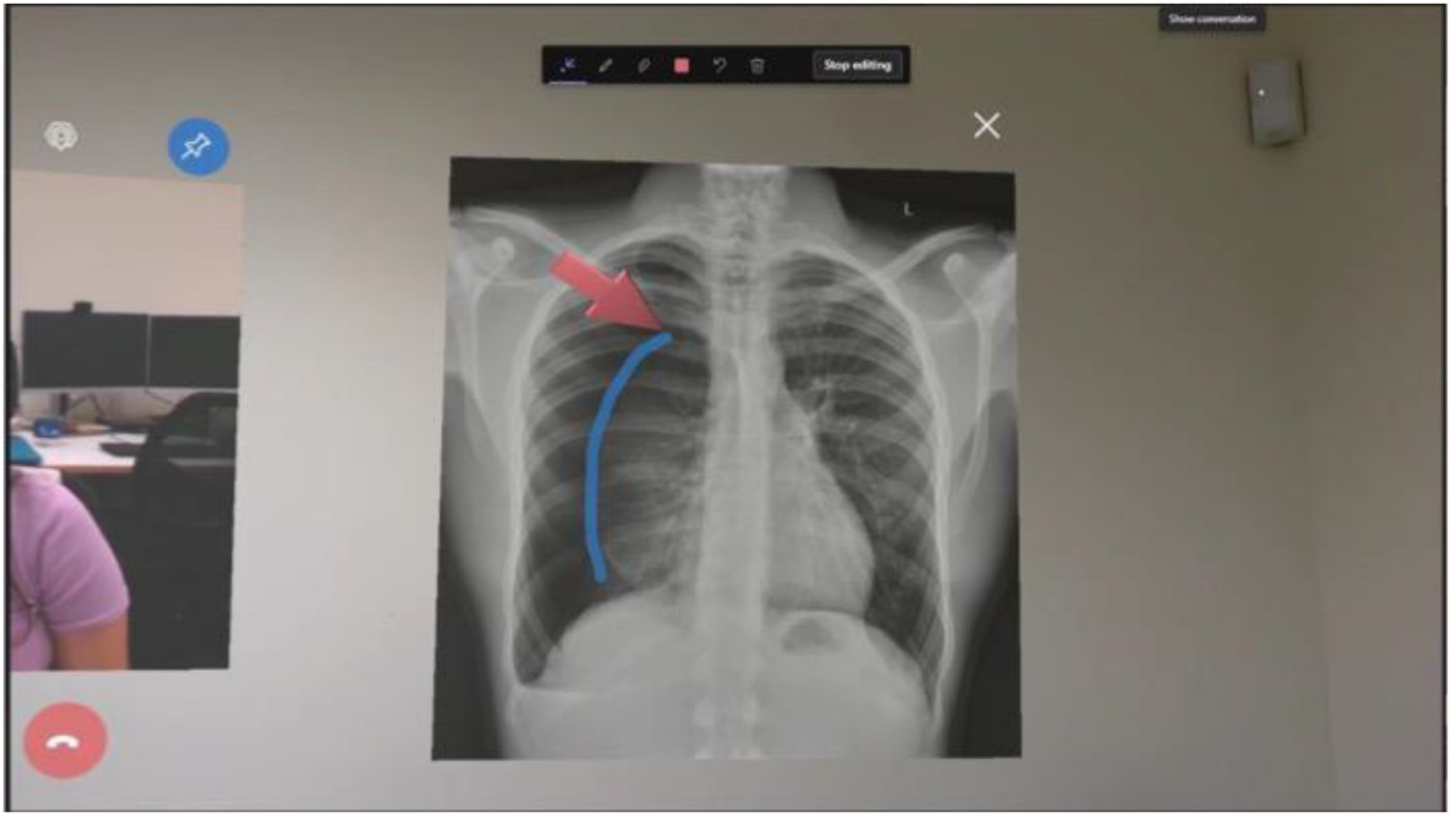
An example of file sharing and annotation used in scenario 4 with the HoloLens 2. The Advising Clinician shared the patient's chest X-ray with the Rural Clinician which appears in three-dimensional space beside the video call which has been pinned to a blank wall. The Advising Clinician has then annotated on the X-ray to educate the Rural Clinician on the pneumothorax and planned location for the needle decompression.
